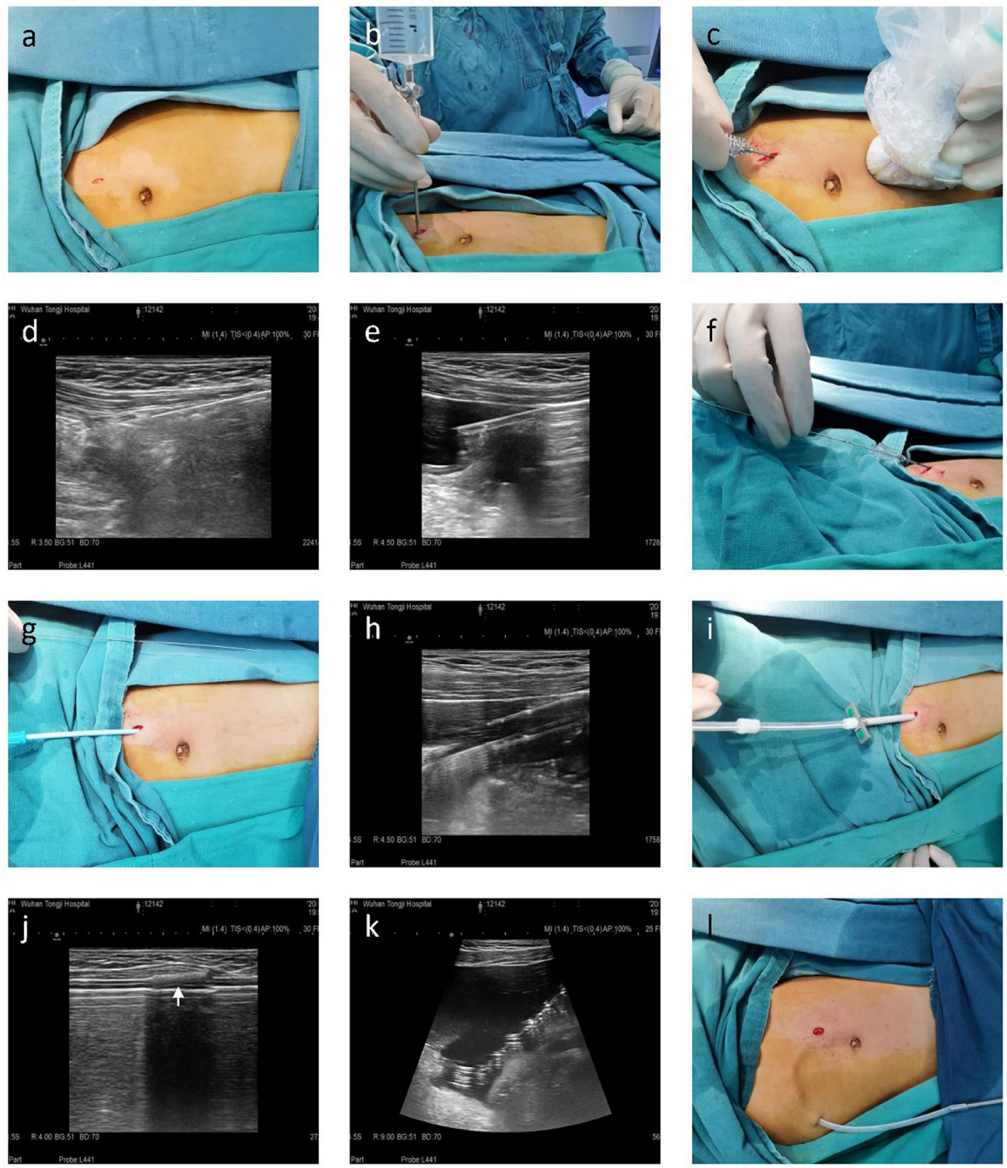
Seldinger placement of a Tenckhoff catheter in a representative pediatric patient in preparation for peritoneal dialysis. (a) A 1 cm linear incision was made, and subcutaneous tissue was blunt separated to reach the anterior sheath of the rectus abdominis. (b) A hydroperitoneum was established using a Veress needle. (c–e) A 18-gauge coaxial biopsy needle was advanced in a caudal direction toward the pelvis at 30–45° from the skin surface using ultrasound guidance. (f) A 0.035-inch guide wire was inserted through the coaxial biopsy needle and directed toward the pelvis. (g,h) 8-French, 12-French, and 14-French introducer sheaths were sequentially placed over the guide wire. (i–k) The PD catheter was introduced over the rigid guide wire within the 16-French pull-apart sheath after removal of the inner sheath dilator. Ultrasound was used to confirm the deep cuff and tip of the PD catheter reached the rectus abdominis muscle and Douglas pouch, respectively. (l) An 8–12 cm subcutaneous passage for the PD catheter was established using a stylet, and the second cuff was 2 cm from the exit.
